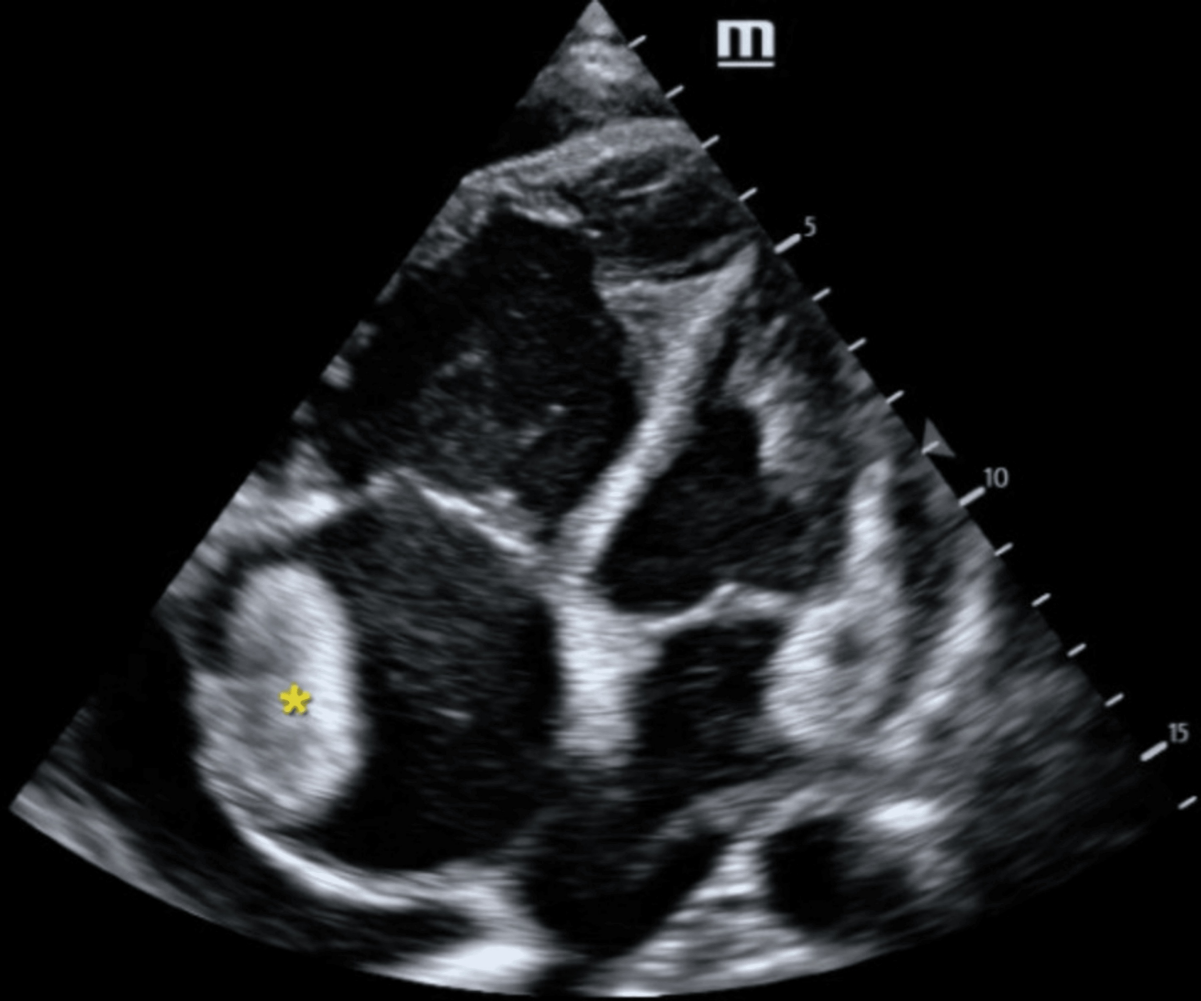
Apical four chamber view demonstrating an isoechoic mass attached to the lateral right atrial wall (yellow asterisk). Right ventricular enlargement is evident.
